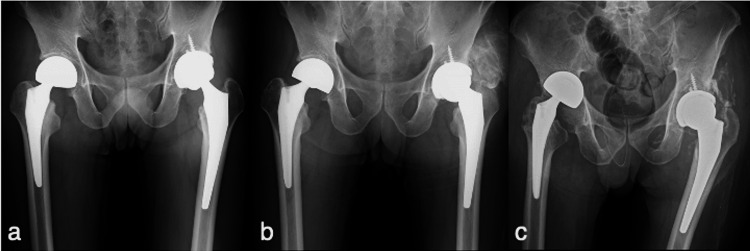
Anteroposterior radiograph. (a) At seven years after left total hip arthroplasty (THA) and three months after right hemiarthroplasty. (b) At 11 years after THA and four years after hemiarthroplasty. (c) At 13 years after THA and six years after hemiarthroplasty.
